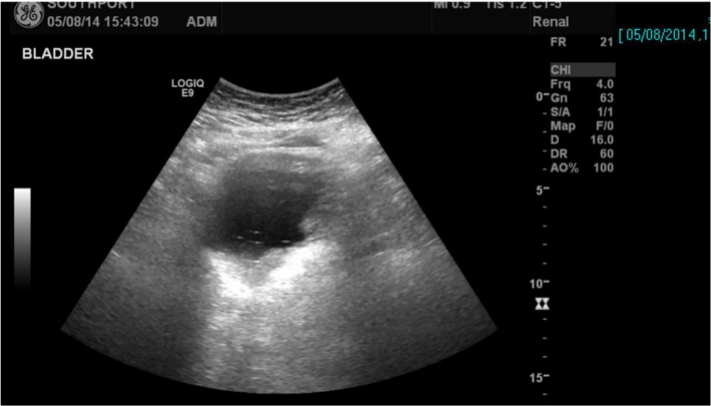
Ultrasound scan of urinary bladder: the balloon of Foley catheter is not seen, but the importance of this finding was not recognised by radiologist or by spinal cord physician.
